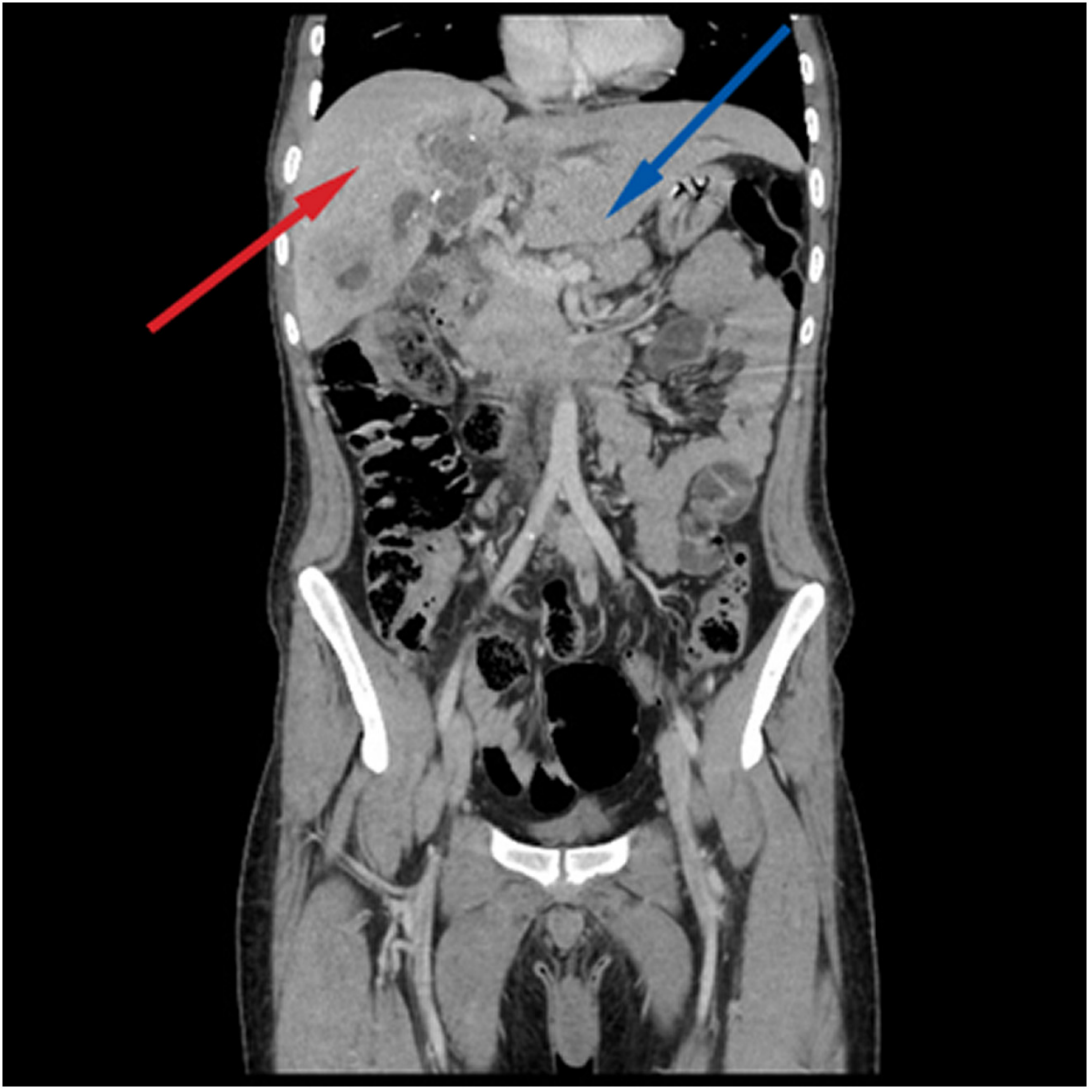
Abdominal CT (coronal) scan demonstrating elongated left lobe of the liver mimicking a beaver's tail (blue arrow) with the normal right lobe of the liver (red arrow); a beaver tail appearance liver. measuring about 15.5 cm with evidence of two occupying low‐attenuation lesions: one in segment IV and one in segment V, measuring approximately 5.5 × 4 cm and 3.5 × 5.9 cm, respectively. These lesions appeared as thick‐walled fluid‐filled lobulated structures with incomplete peripheral coarse calcifications surrounded by an ill‐defined faint heterogeneous density area and adjacent capsular retraction.
